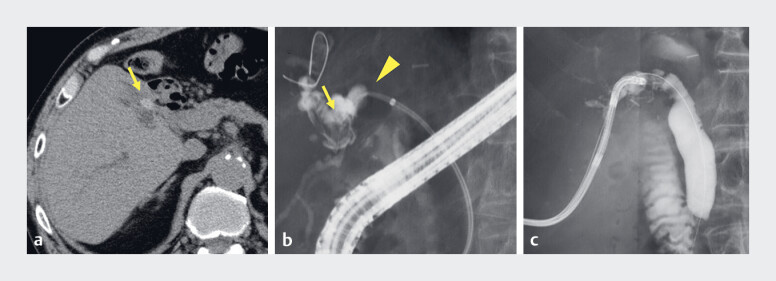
Computed tomography and cholangiography images.
